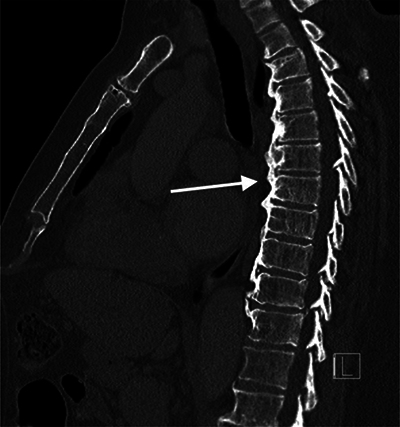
CT thorax sagittal images in bone window.
